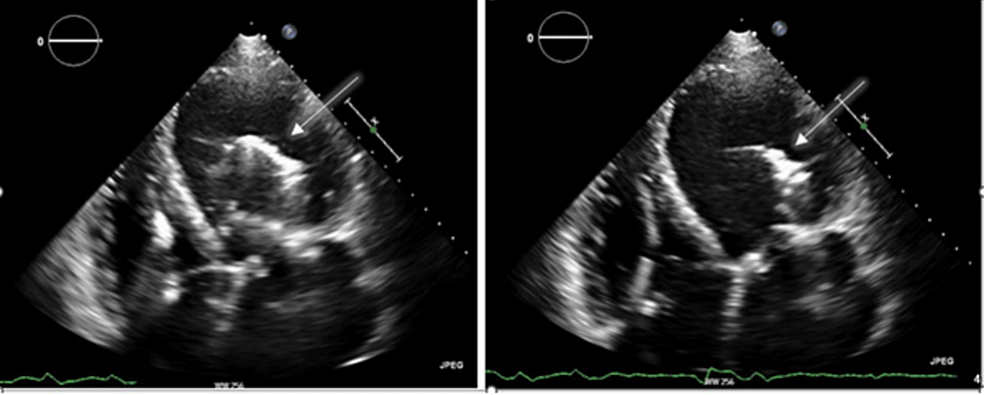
A four-chamber apical view TTE showing an echo dense mass (white arrow) representing the IABP in the LV TTE: trans-thoracic echocardiogram; IABP: intra-aortic balloon pump; LV: left ventricular
